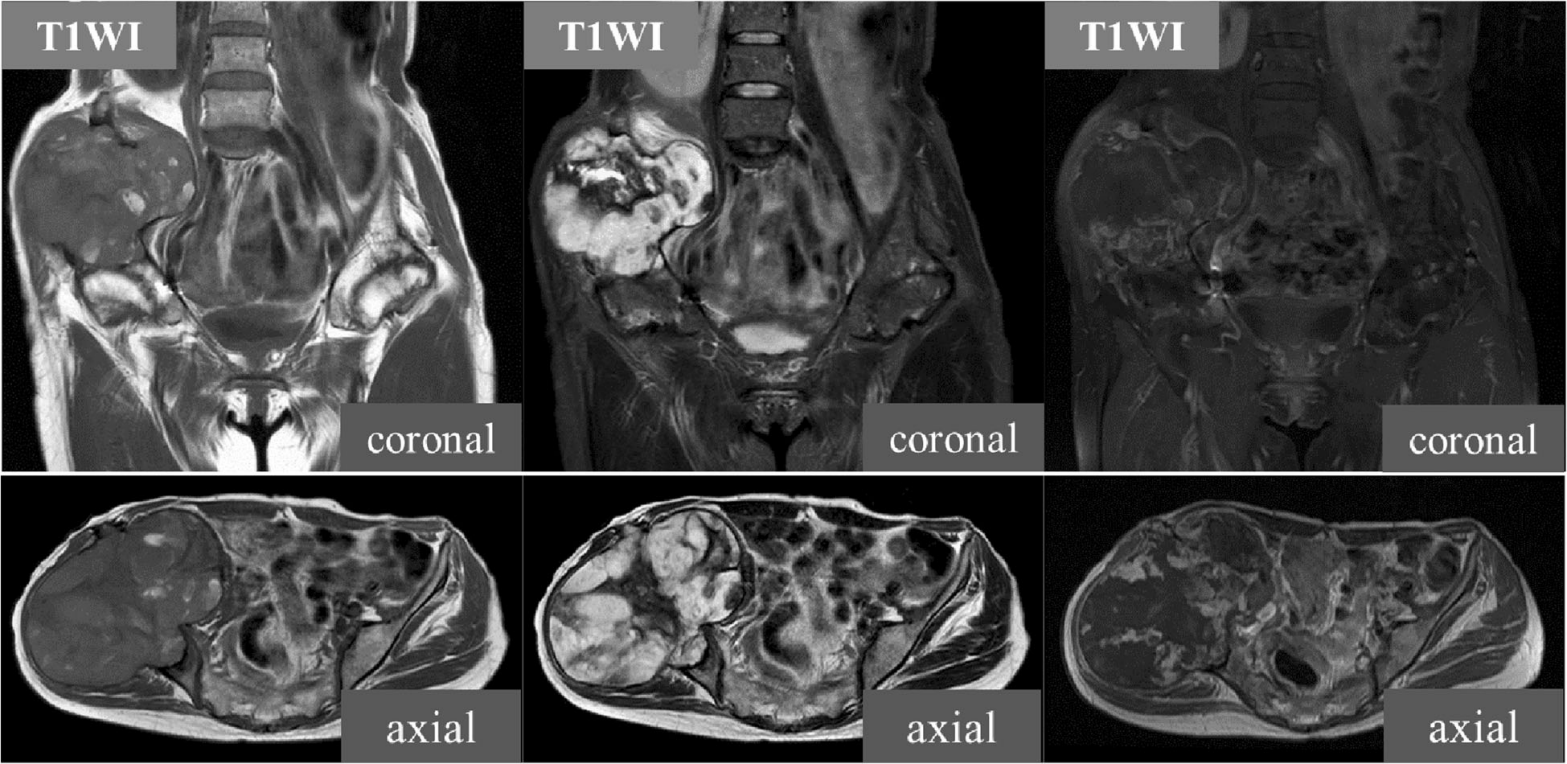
Magnetic resonance imaging of the ilium. The lesion of the right ilium showed predominantly isointense or high signals on T1-weighted images, and a mixture of low and high signal intensities on T2-weighted images. The heterogeneous enhancement of the mass following the intravenous injection of gadolinium-diethylenetriaminepenta-acetic acid is visible on the T1-weighted image
